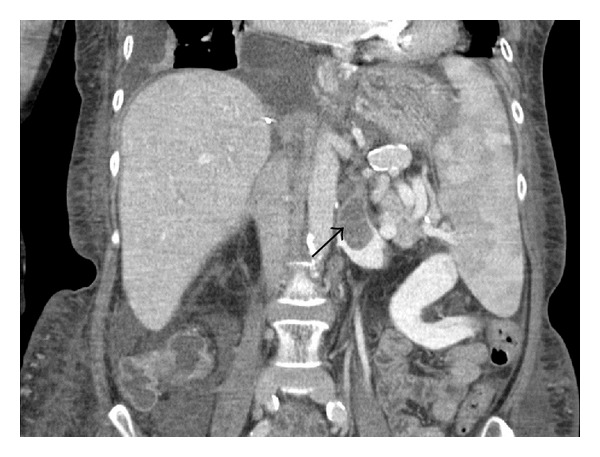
Postoperative CT imaging (day 47) showing in axial form the thrombosis of the left renal vein (arrow).
